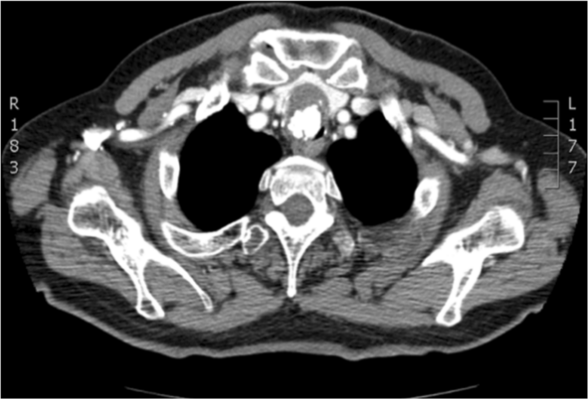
CT image demonstrating the tracheal lesion at presentation
